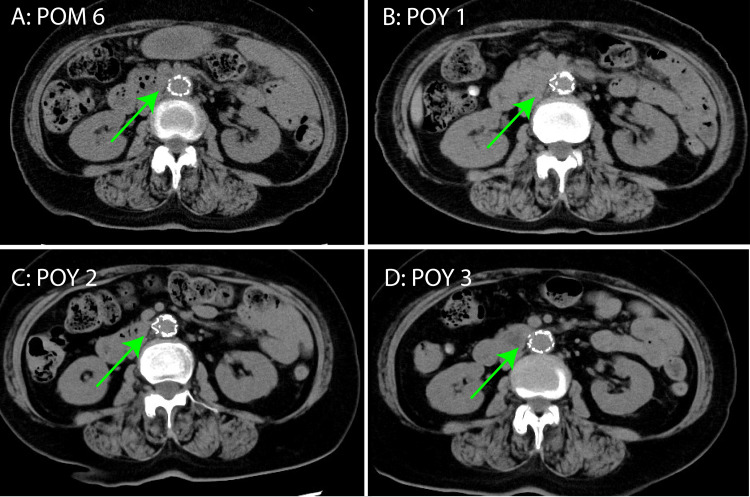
Postoperative enhanced computed tomography images (A) Postoperative month 6 (POM 6). (B) Postoperative year 1 (POY 1), (C) POY 2, and (D) POY 3. Periaortic air or signs suggestive of infection were not evident around the abdominal aorta (green arrow).
